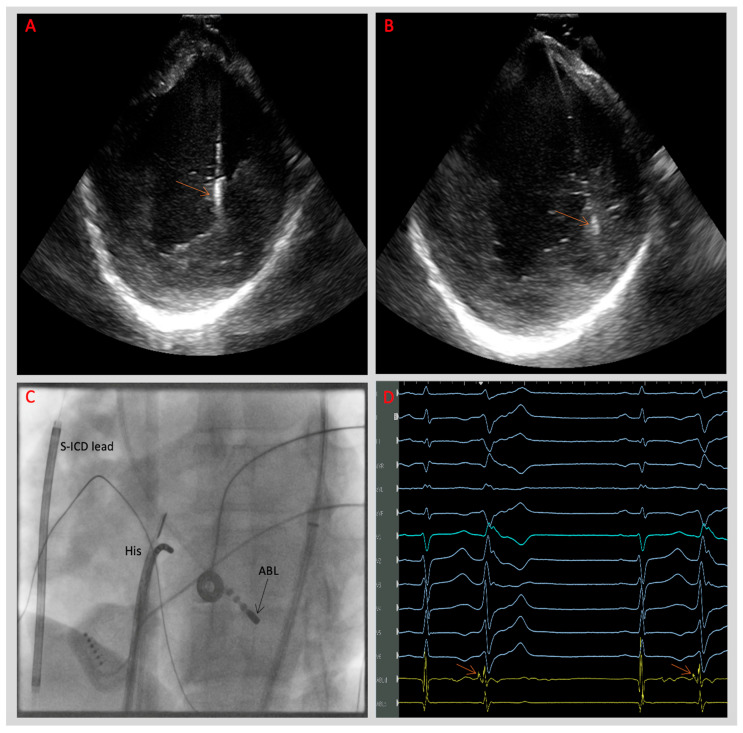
(A,B) Intracardiac echocardiography (ICE) views of the left ventricle demonstrating ablation catheter contact (arrows) with posteromedial papillary muscle (PM) during radiofrequency (RF) energy delivery in a patient with AMVPS. (C) Fluoroscopy in the same patient demonstrating an ablation catheter tip (black arrow, ABL) at the PM level. (D) Electrogram demonstrating the site of successful ablation in a patient with AMVPS with frequent PVCs originating from posteromedial papillary muscle (RBBB morphology with superior axis) preceded by Purkinje potentials (arrows on the ablation catheter [ABL] channel). (His: Hexapolar catheter at the His bundle level, S-ICD: subcutaneous implantable cardioverter defibrillator).
